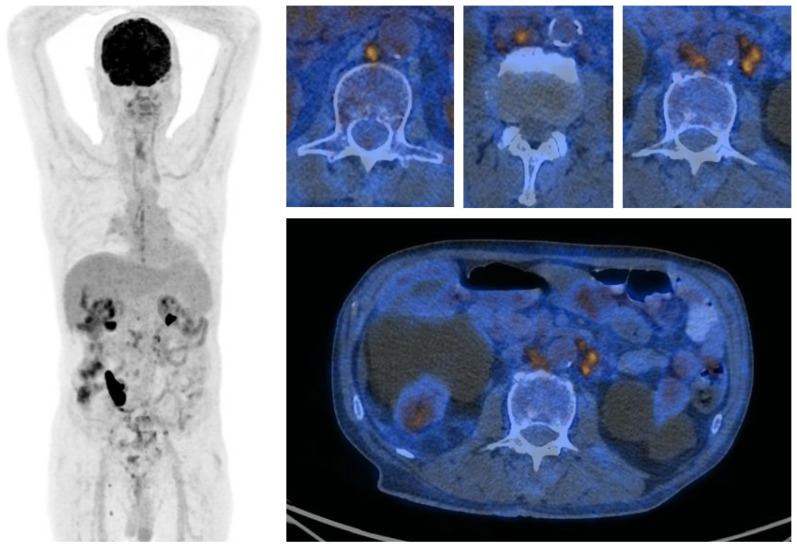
[^18^F]FDG PET/CT, a 74-year-old patient with bladder cancer after cystectomy. A dose of 4 MBq/kg was administered, and imaging was performed approximately 60 min after tracer injection. Recurrence in retroperitoneal lymph nodes with high tracer uptake. Increased tracer activity in the sternum is associated with another previous surgery.
